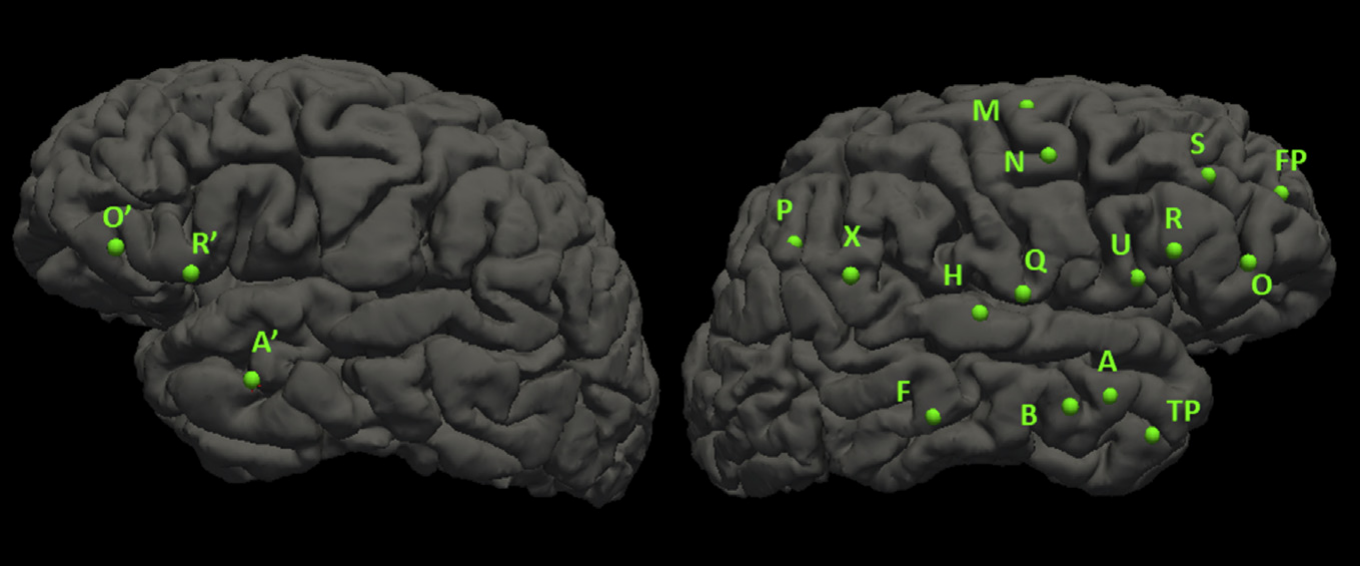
Surface map of implanted electrodes, reconstructed from raw MRI data. Implantation was heavily weighted to the right hemisphere based on the Video EEG evaluation which only demonstrated right sided seizures.
